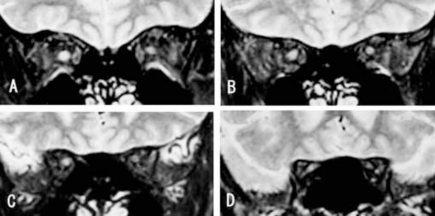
Typical MR image of a case with right ON. A: 5mm, B: 10mm, C: 15mm and D: 20mm behind eye.
